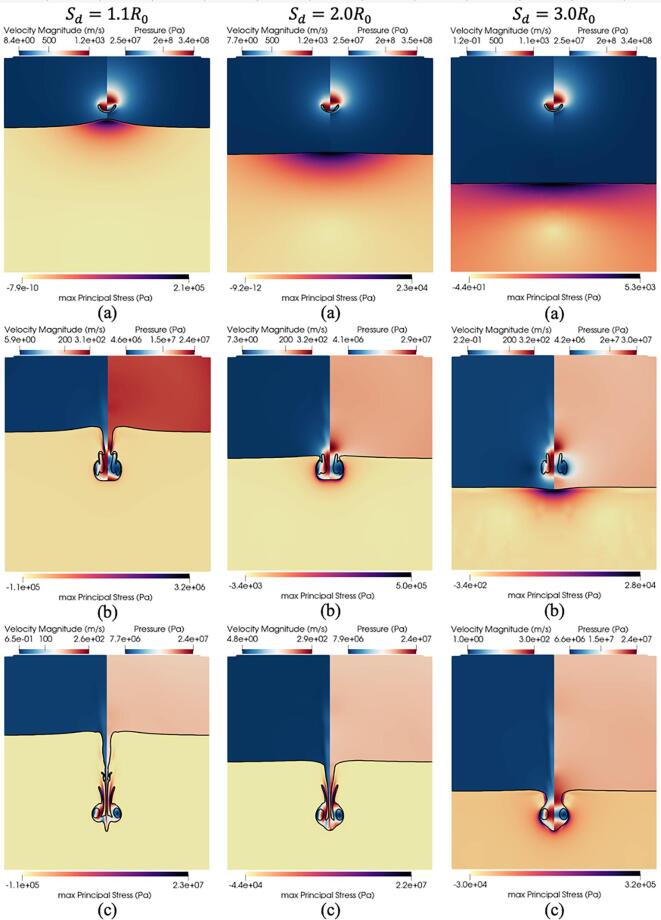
Effect of the standoff distance. Ultrasound-induced collapse of an air bubble of $R_{0}=10\mathrm{\mu m}$ near bileduct at t=0.19μs, t=0.31μs, and t=0.44μs for standoff distances $S_{d}=1.1R_{0}$, $S_{d}=2.0R_{0}$ and $S_{d}=3.0R_{0}$. The black isosurface separates the three materials and is defined by $\alpha_{k}=0.5.$ Upper left hand: contour of velocity magnitude. Upper right hand: contour of pressure. Bottom half: contour of the maximum principal stress.
